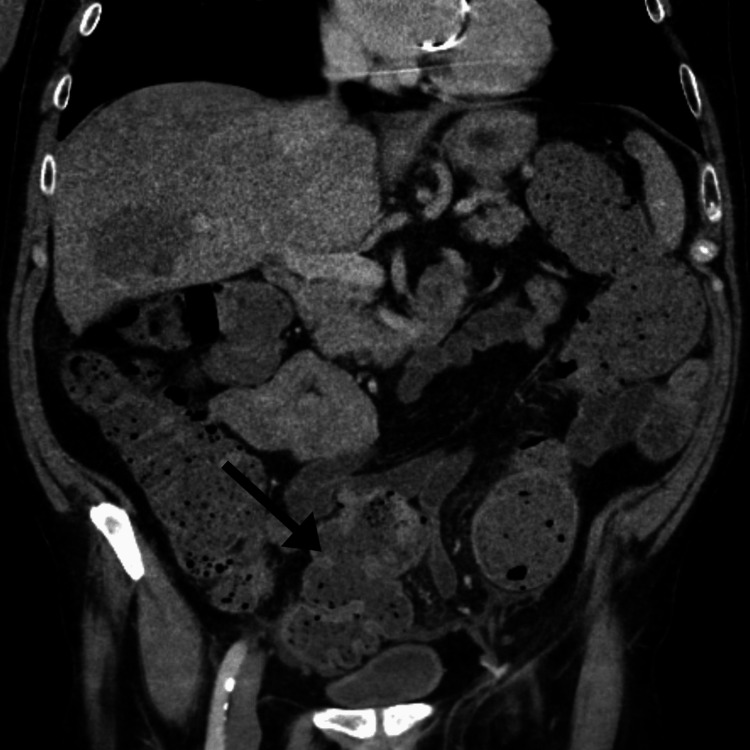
Computed tomography scan in coronal view demonstrating moderate dilation of the sigmoid colon, surrounding inflammatory changes, and luminal wall thickening.
